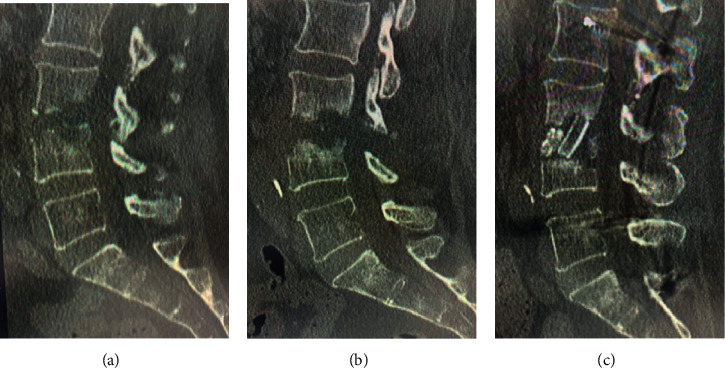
A 70-year-old female was diagnosed with L3/L4 infectious spondylitis and underwent endoscopic debridement, although the pain was increased postoperatively. She underwent anterior reconstruction surgery with iliac autograft. (a) The preoperative sagittal CT scan view showing severe osteolytic changes at the L3/L4 level. (b) The post-FED sagittal CT scan view showing an exacerbated defect of the vertebra. (c) The post-open surgery sagittal CT scan view; the iliac strut bone was grafted.
